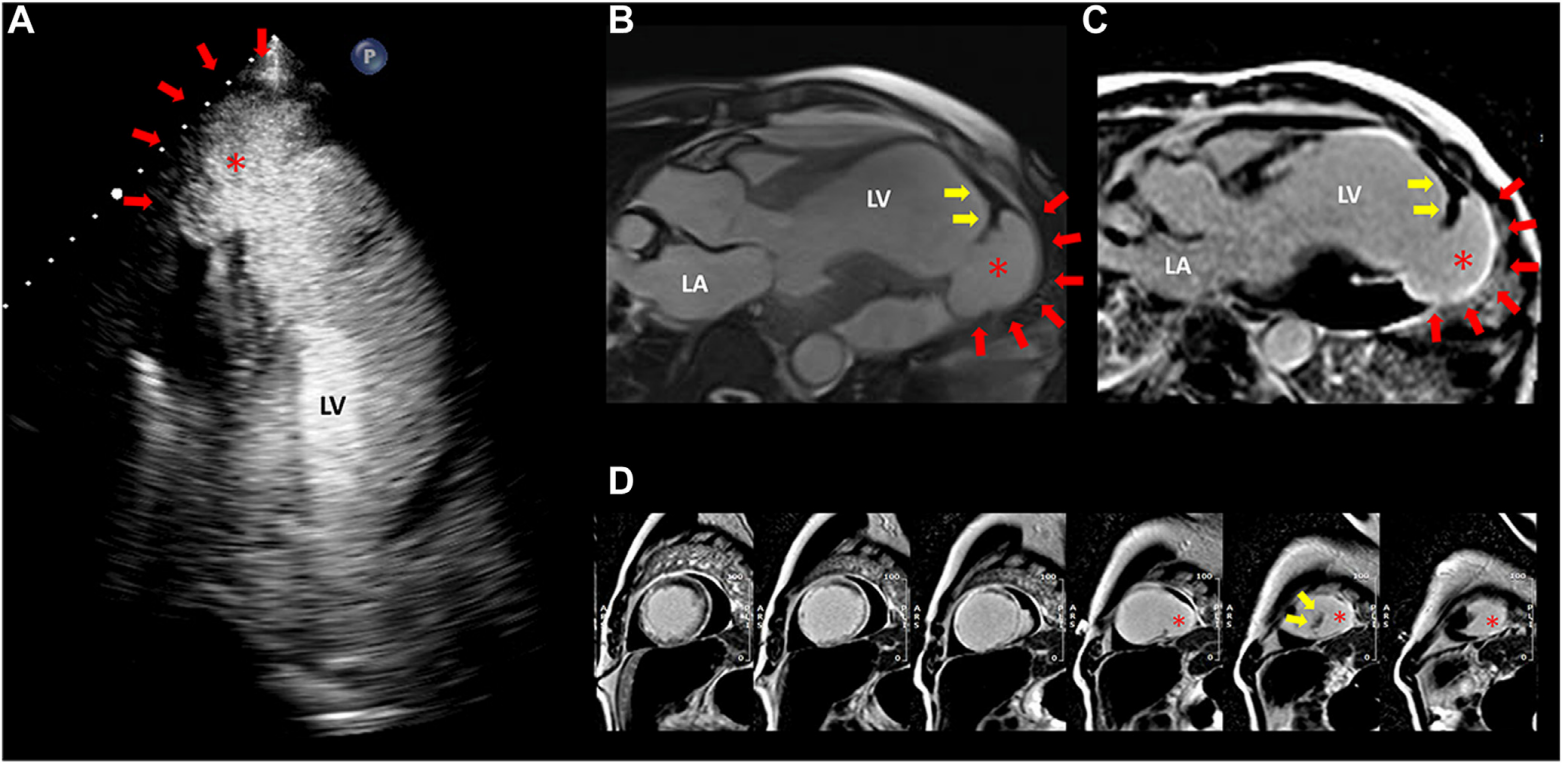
Follow-up transthoracic echocardiogram and cardiac magnetic resonance imaging. (A) Apical 3-chamber view on the transthoracic echocardiogram with an ultrasound-enhancing contrast showing a morphologic change of the left ventricular (LV) aneurysm suspicious for pseudoaneurysm (asterisk and red arrows). The imaging hallmarks of pseudoaneurysm include a saccular-shaped structure with a narrow neck and an abrupt transition in wall thickness and angulation at its origin. (B) Three-chamber steady state free precession cine imaging demonstrating pseudoaneurysm formation (asterisk and red arrows) in the apical inferolateral segment, and remnant LV thrombus (yellow arrows) with indexed LV end-diastolic and end-systolic volumes of 232 mL/m^2^ and 184 mL/m^2^, respectively. (C,D) Late gadolinium enhancement imaging demonstrating the pseudoaneurysm (asterisk and red arrows) and remnant thrombus (yellow arrows); (C) 3-chamber view; (D) short-axis views from mid-ventricular to apex). LA, left atrium; (in figure) LV, left ventricle.
